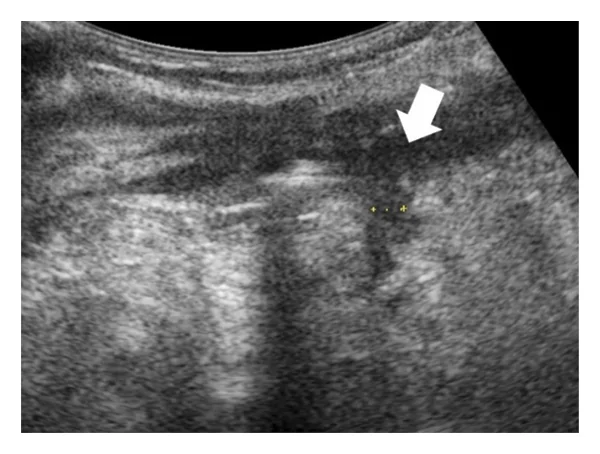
Follow‐up abdominal ultrasonography after initial treatment demonstrating shrinkage of the abdominal wall abscess and marked reduction of the fistulous tract (arrow).
